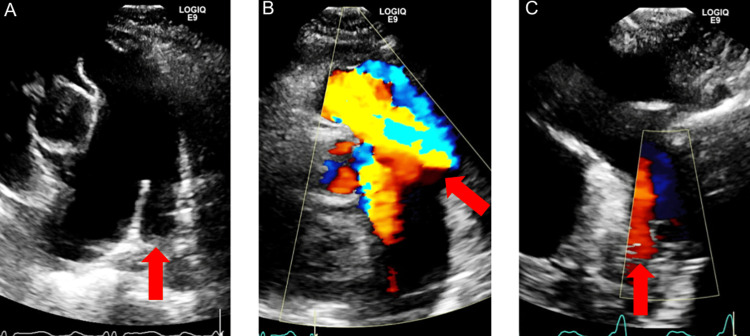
A: Parasternal short-axis view of the pulmonary artery showing a PDA (indicated by the red arrow). B: Arrow shows the flow into the pulmonary artery from the aorta. C: Suprasternal view showing color Doppler of the aortic arch and descending aorta with diastolic flow reversal (indicated by the red arrow).
